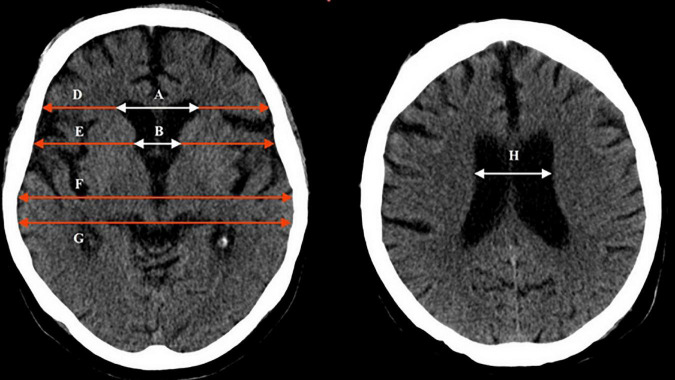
Brain computed tomography, indicating the different indices used in this study and how they were calculated: Bifrontal index = maximum width of frontal horns/skull width at the same level = A/D. Evans index = maximum width of frontal horns/skull width at the level of the third ventricle = A/F. Bicaudate index = minimum width of lateral ventricles/skull width at the same level = B/E. Ventricular index = minimum width of lateral ventricles/maximum width of frontal horns = B/A. Cella media index = maximum width of the skull/width of lateral ventricles = G/H.
